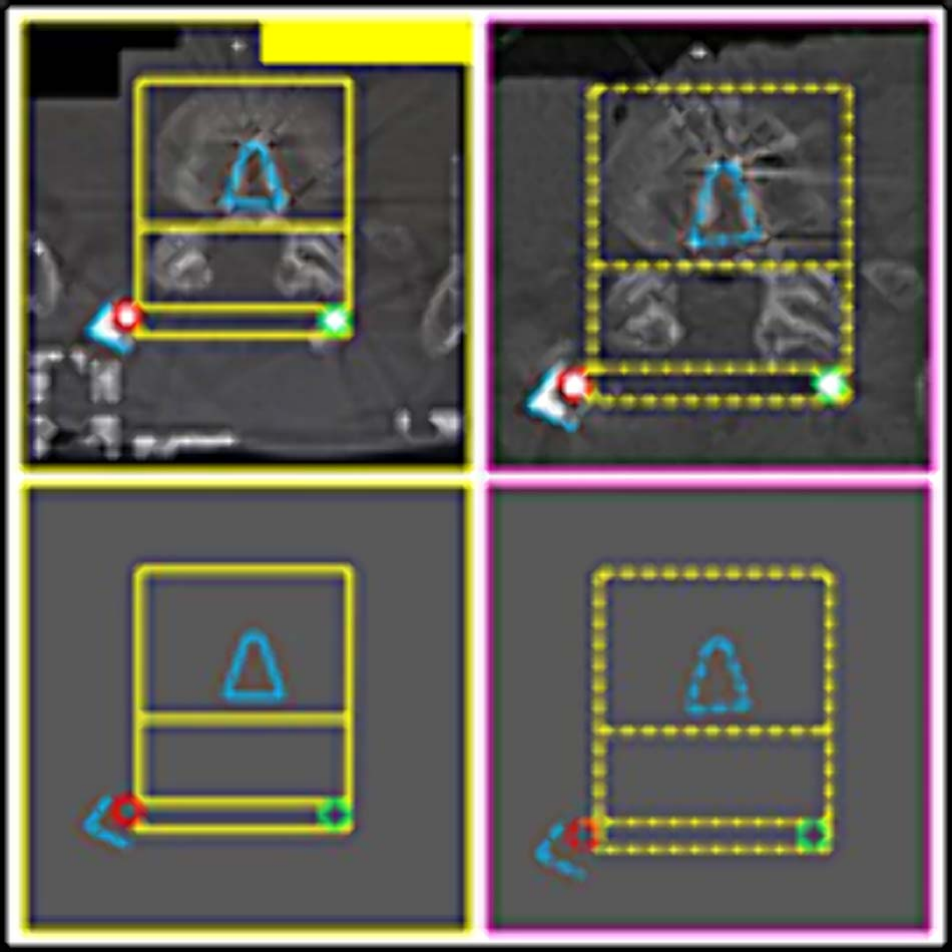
L5 vertebral body. Upper panels, comparison between the axial slice images of the antemortem (left) and postmortem (right) CT scans. Lower panels, respective concordant templates.
